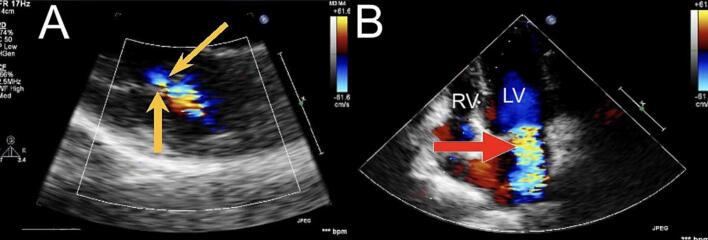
Pre-operative two-dimensional echocardiogram demonstrating severe mitral regurgitation. (A) Long-axis view showing a 0.7 cm vena contracta indicated by yellow arrows. (B) Apical four chamber view showing regurgitant flow (red arrow) indicating severe mitral stenosis. RV: right ventricle, LV: left ventricle. (For interpretation of the references to color in this figure legend, the reader is referred to the web version of this article.)
